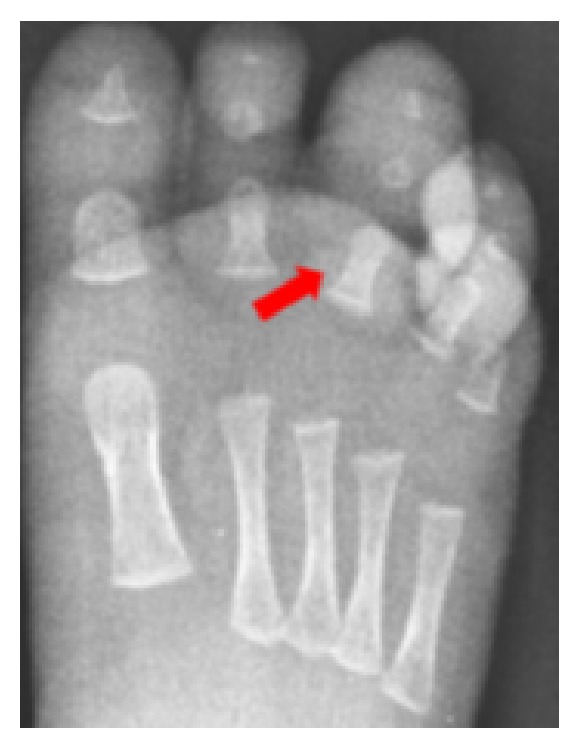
Plan X-ray showing bone erosion (arrow).
